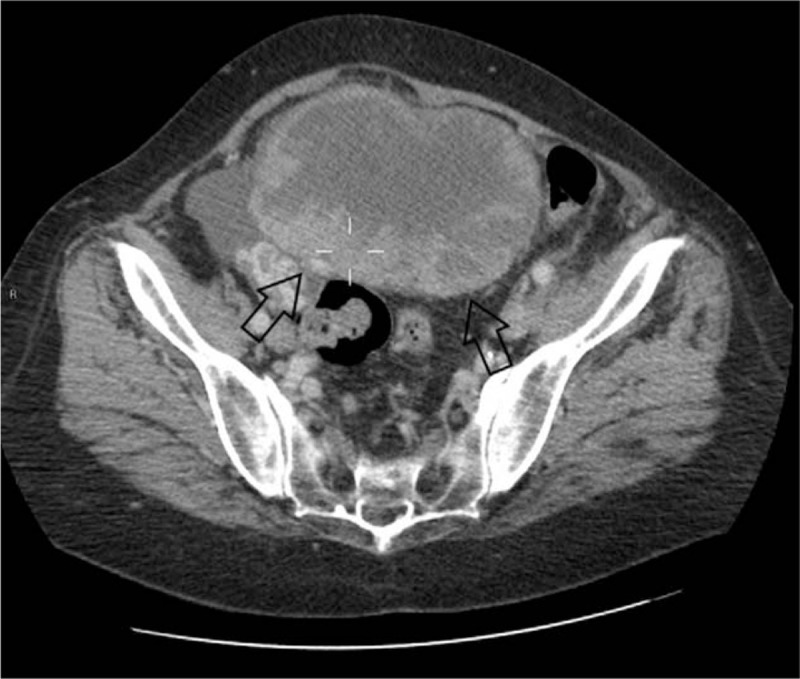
Computed tomography (CT) scan. Presence of peripheral enhancement (black arrows).
